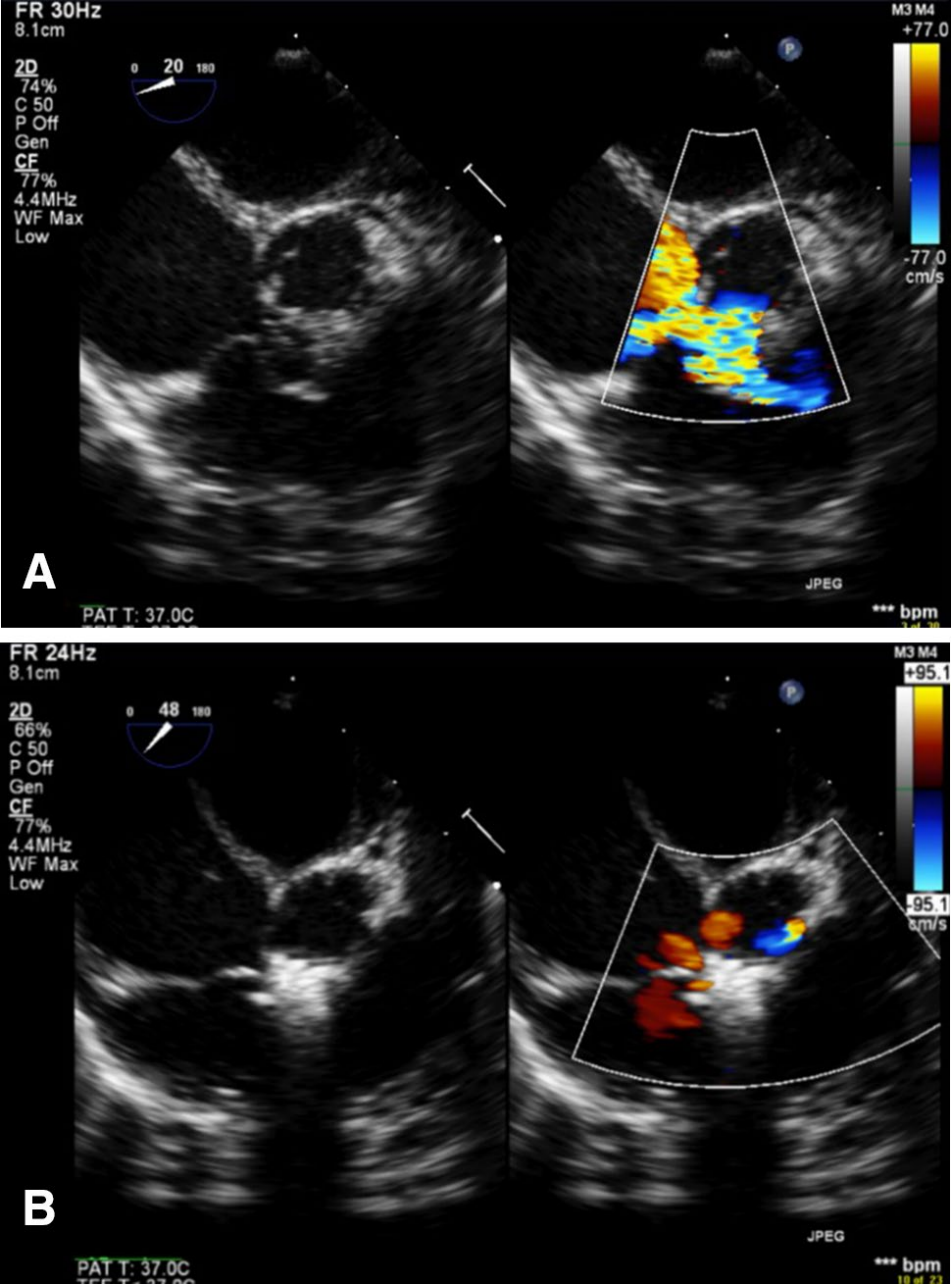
Transoesophageal echocardiography. A Demonstrates 2D and colour mode for a PMVSD with a moderate degree of tricuspid regurgitation pre-operatively. B Display the device in situ with mild degree of tricuspid regurgitation
